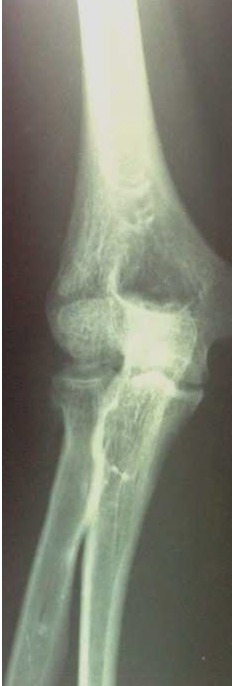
Radiographie de face du coude droit
